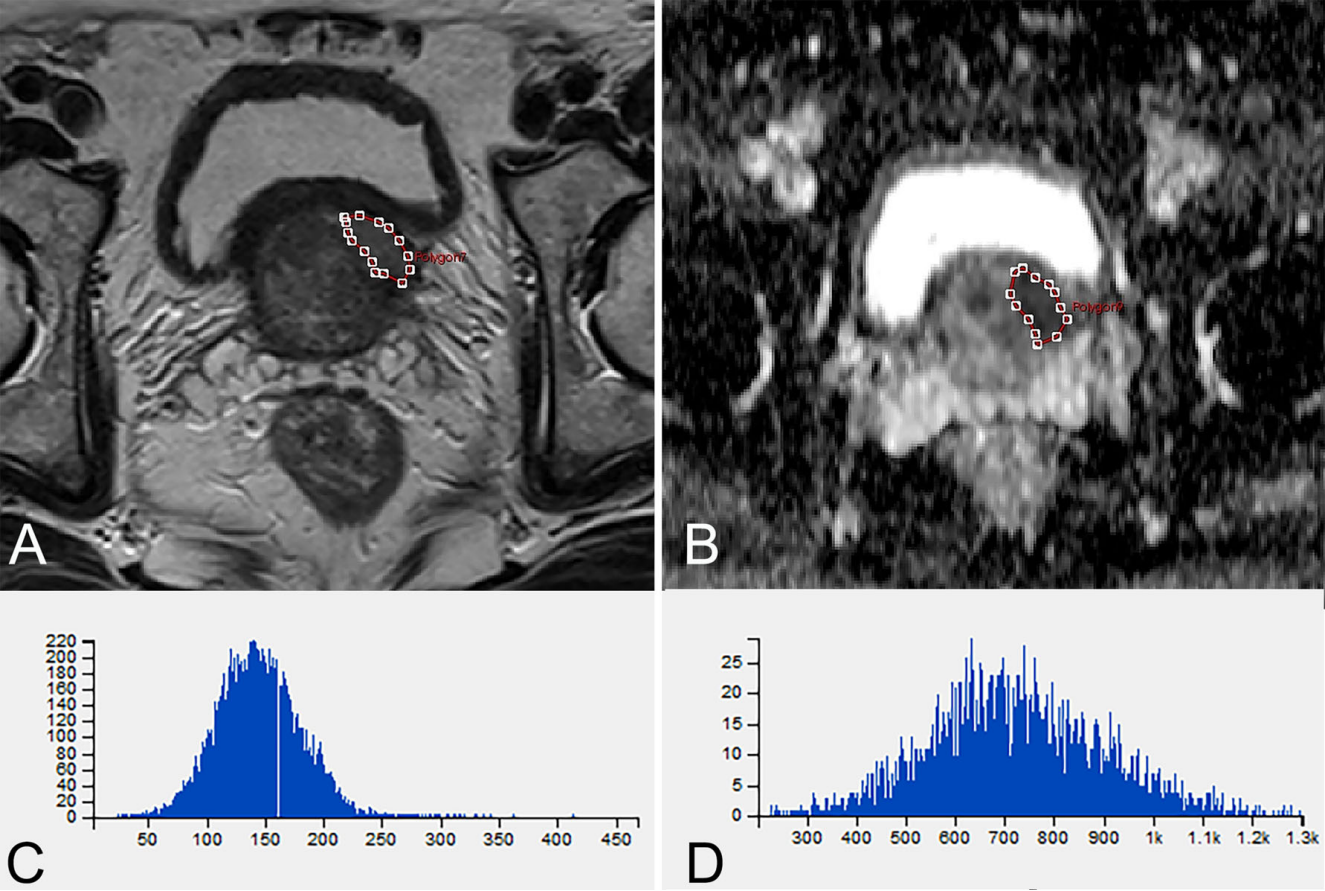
A 66-year-old man with Gleason score 4 + 3 = 7 lesion. A T2-weighted MR image shows ROI of lesion (red) manually drawn for transition zone. B ADC mapping image shows ROI of lesion (red) manually drawn for transition zone. C Histogram of A. D Histogram of B
